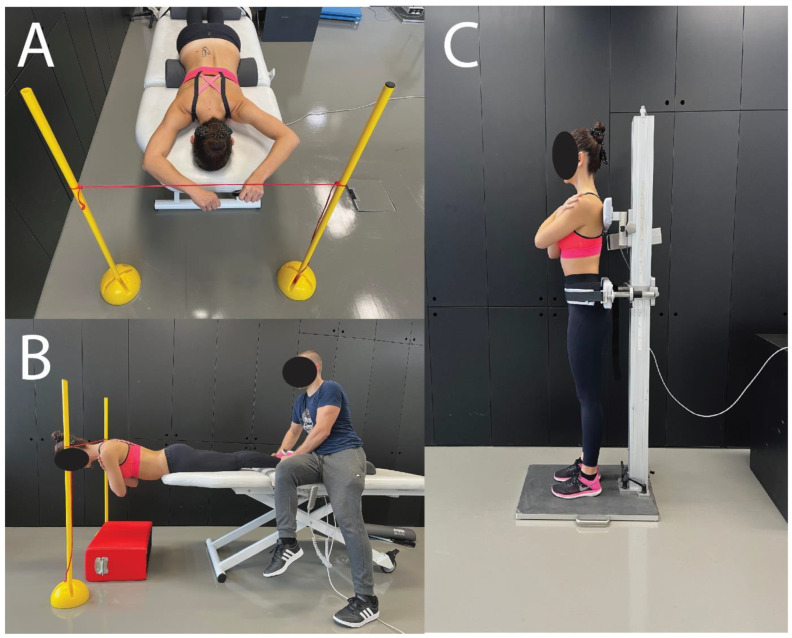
Participant positioning for low-level (A) and high-level contractions (B) and set-up for isometric trunk extension force measurements (C). Ultrasound measurements are not shown in figures A and B to allow clearer representation of the participant positions.
